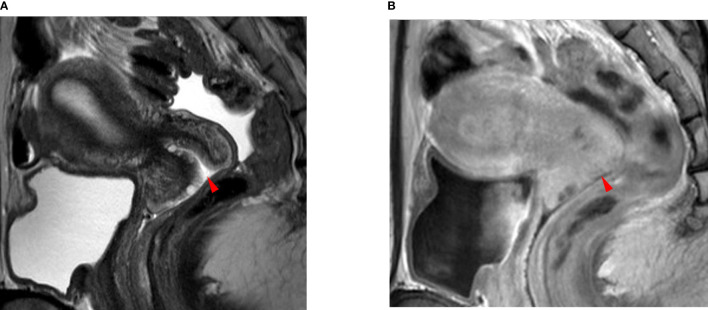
A 35-year-old woman with squamous cell carcinoma. (A) The T2-weighted sagittal MR image shows no focal lesion in the cervix. The red arrowhead indicates the external OS of the uterine cervix. (B) The delayed contrast-enhanced sagittal MR image shows no residual cancer in the cervix. The red arrowhead indicates the external OS of the uterine cervix. The pathologic report confirmed no residual cancer in the resected uterus. There was also no invasion of the lymphovascular space, vagina, parametrium, or lymph node metastasis.
